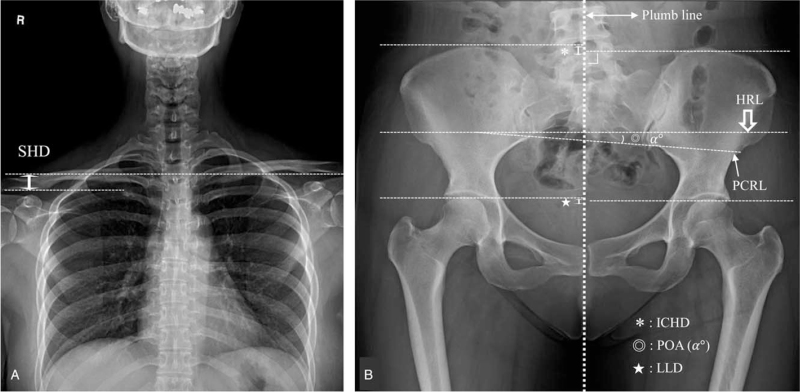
Evaluation of radiographic parameters for spinopelvic imbalance measurement on coronal image. The radiographic parameters included (A) shoulder height differences (SHD, double big arrow), (B) iliac crest height differences (ICHD, asterix), leg length discrepancy (LLD, star), pelvic oblique angle (POA) indicates angle (double circle, @) between horizontal line (HRL, big open arrow) and pelvic coronal reference line (PCRL, narrow arrow).
